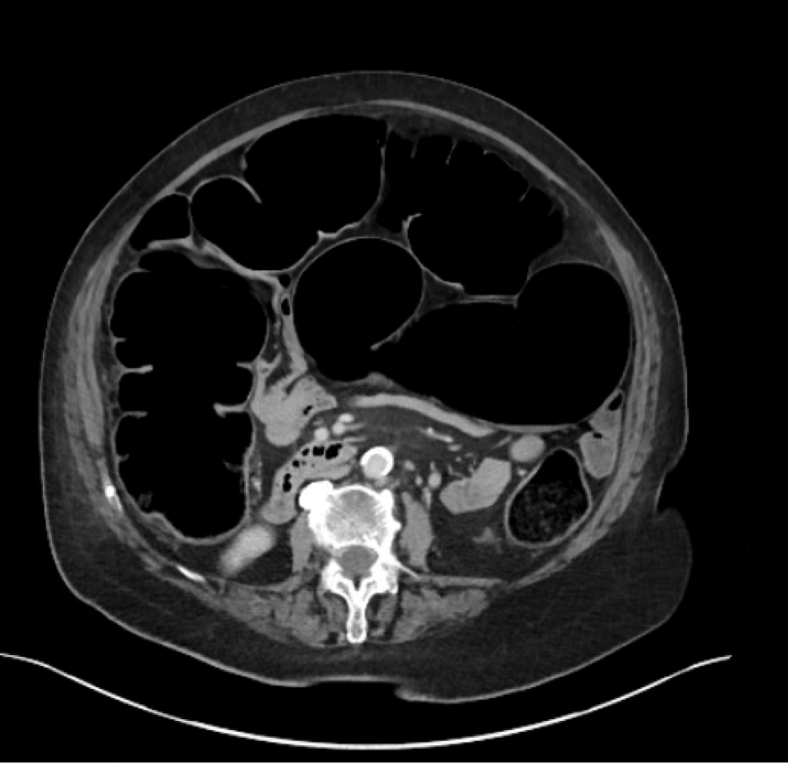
Axial computed tomography scan revealing marked distention of large bowel gas-filled with air-fluid levels and normal appearing appendix.
